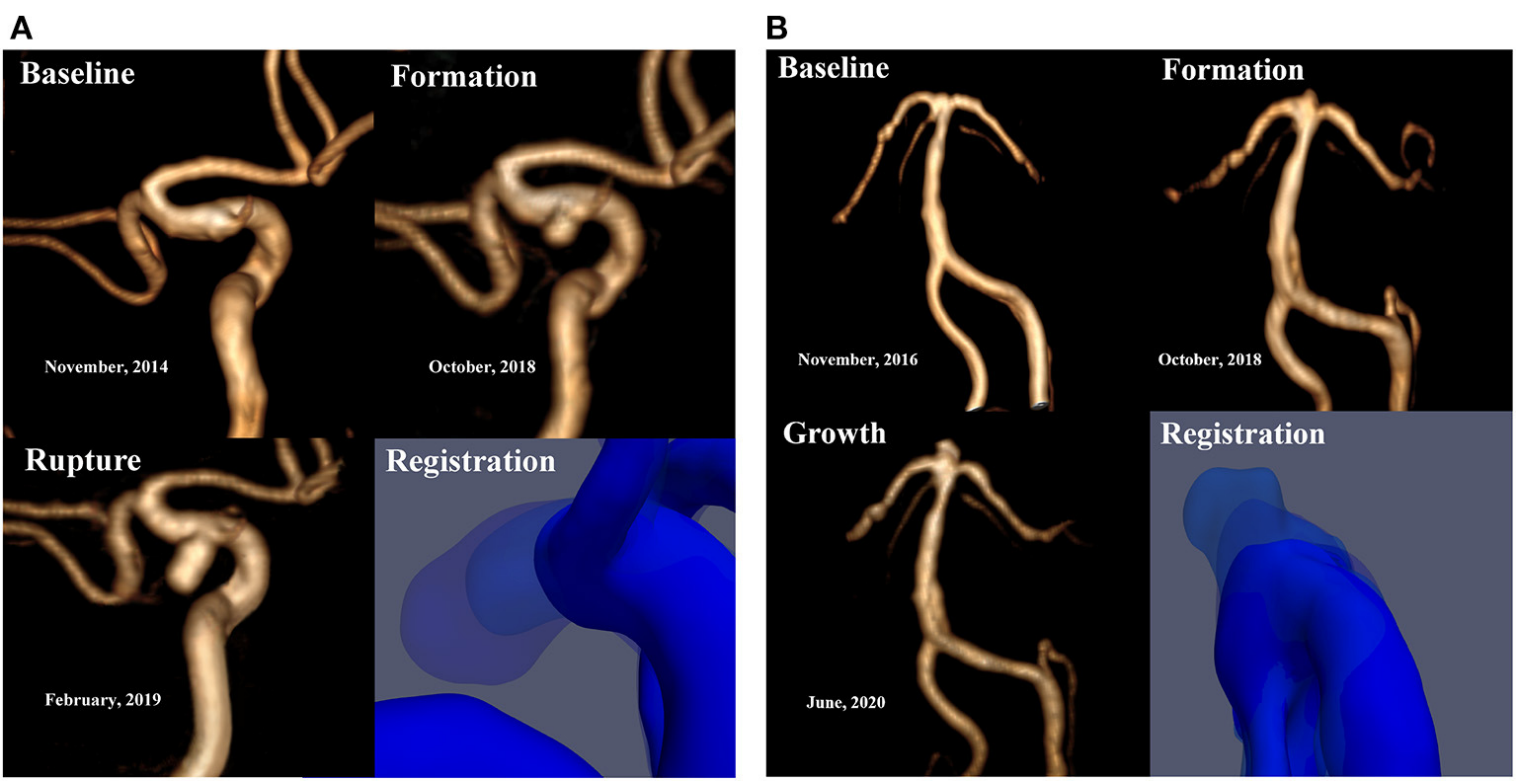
(A) Patient 1 is a 56-year-old female. No significant abnormalities were observed by the baseline MRA. MRA reexamination about 4 years later showed a newly formed saccular aneurysm with a diameter of 3.2 mm in the left posterior communicating artery. CTA showed that the aneurysm had significantly increased in size, reaching a diameter of 6.9 mm. (B) Patient 2 is a 47-year-old female. No significant abnormalities were observed by the baseline MRA. A newly formed basilar tip aneurysm and aneurysmal enlargement were observed in the subsequent MRA examinations at follow-up (from 2.1 to 3.8 mm in diameter). Registration of 3D surfaces with different degrees of transparency showed changes on continuous follow-up angiography.
